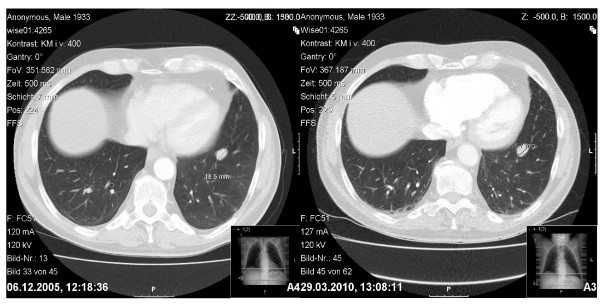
Transversal CT scans of the thorax from the years 2005 (left) and 2010 (right), showing a solid tumor mass in the left inferior lobe of the lung with almost no progress in size. The tumor had a diameter of approximately 1.7 cm in both 2005 and 2010.
